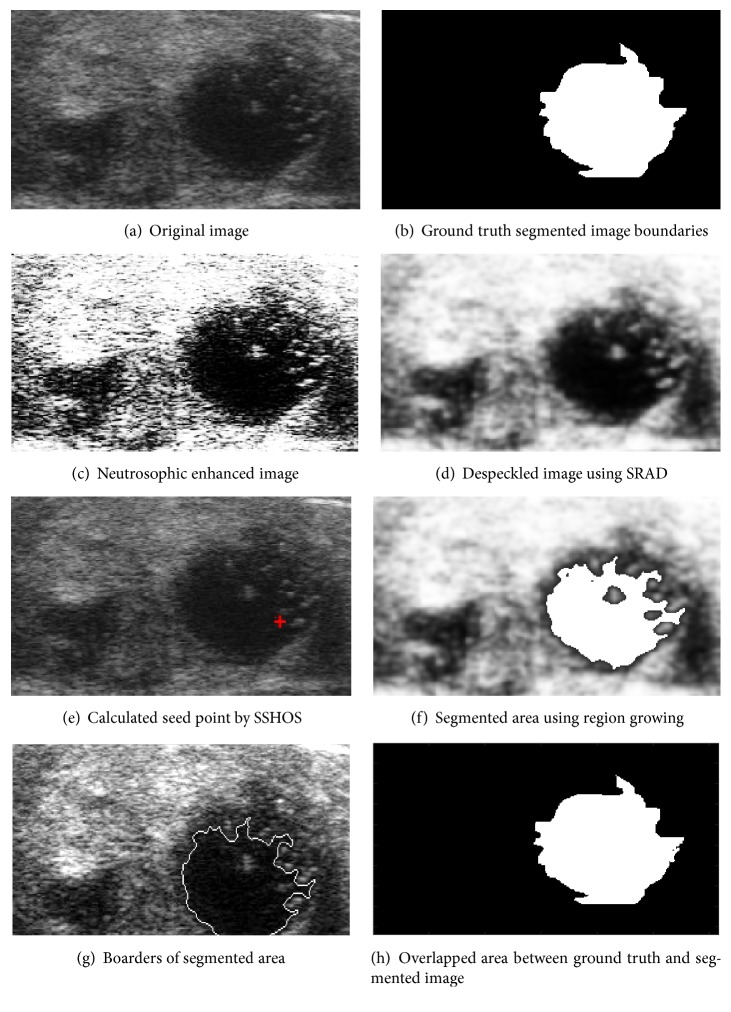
Ultrasound image (image 48B).
